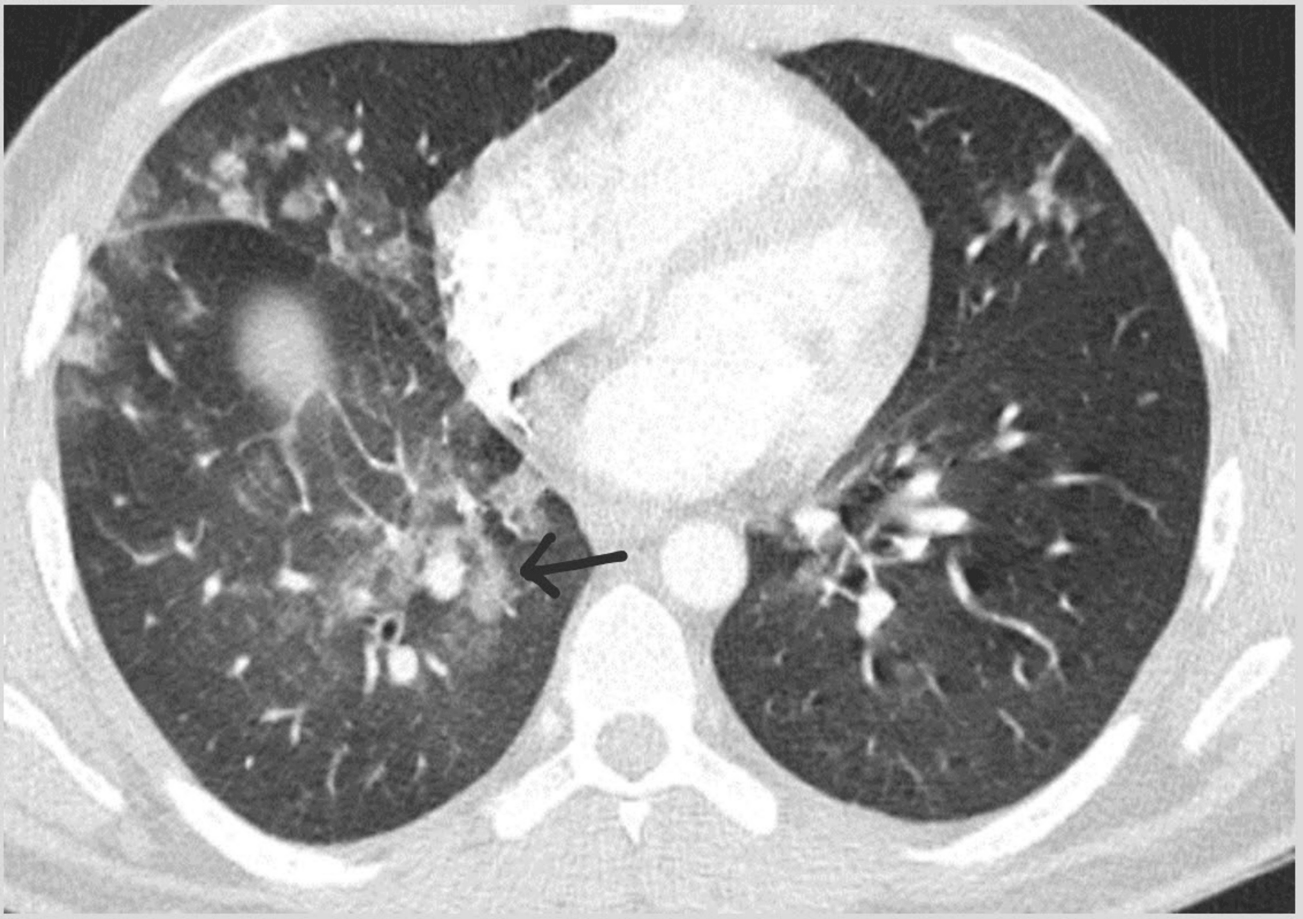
Chest CT conducted in 2023 showing infective changes in lung tissues.
